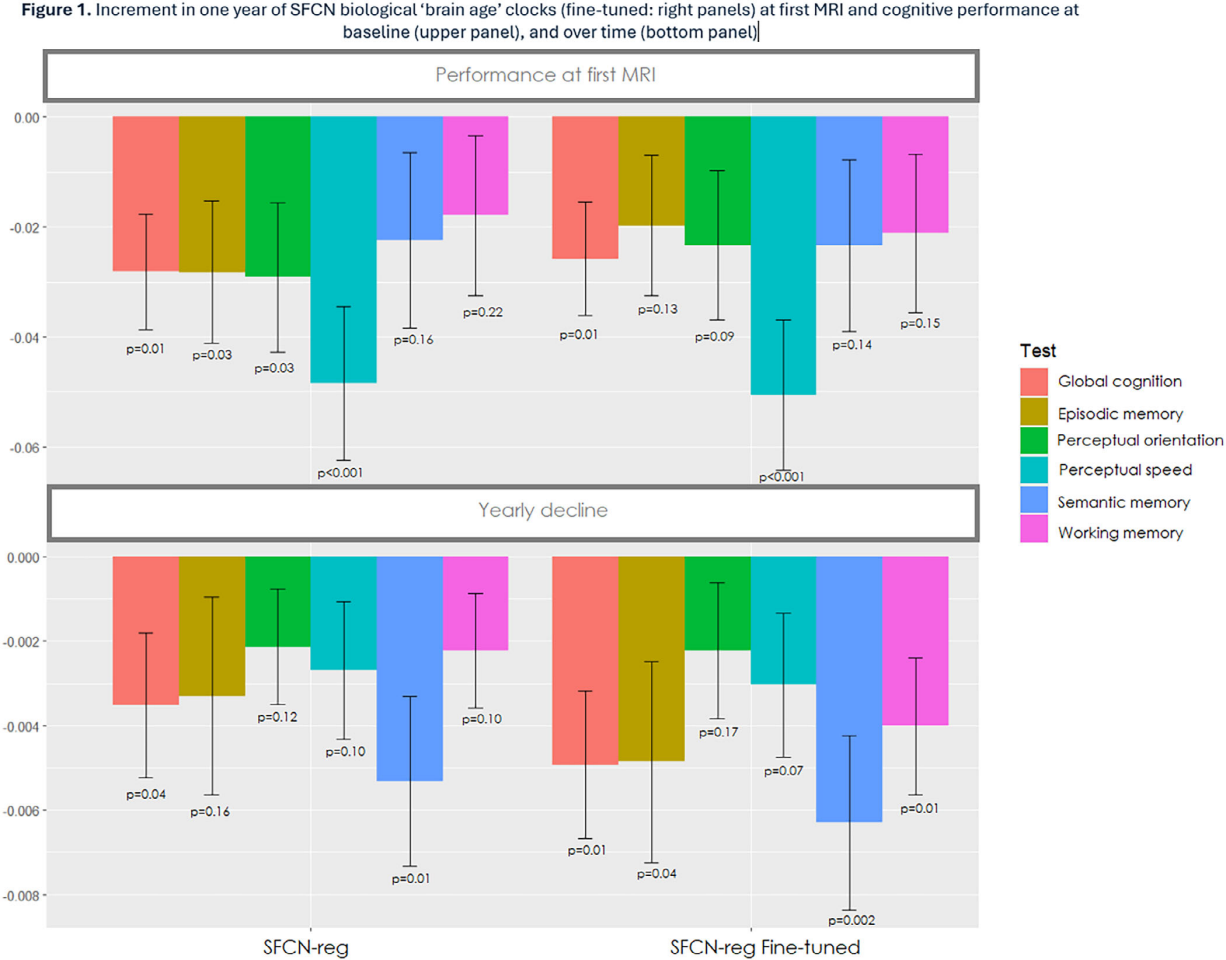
# MRI‐derived biologic clocks and cognitive aging in a sample of older Latino adults
